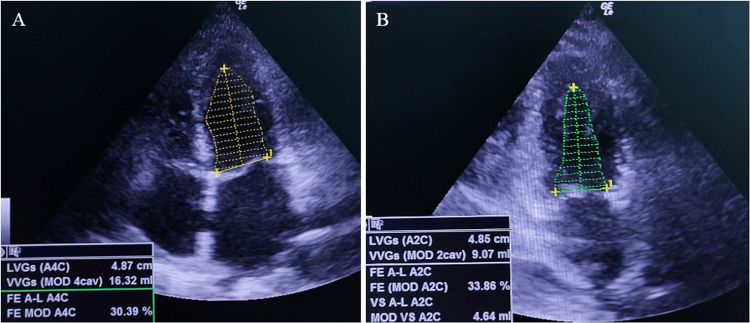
Transthoracic echocardiography was performed on the third postoperative day (A) Four-chamber view demonstrating severe left ventricular dysfunction with markedly reduced contractility across all segments of the left ventricle. (B) The two-chamber view, providing a detailed assessment of the left ventricle, further highlighting the depressed myocardial function. LVEF: 32%. LVEF: left ventricle ejection fraction.
